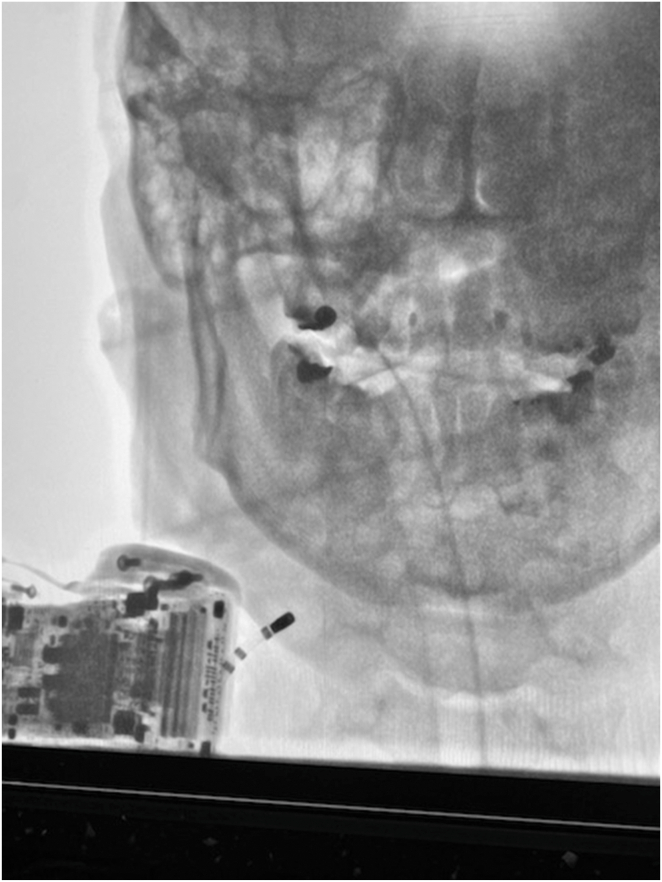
Ultrasound assessment of vagus nerve position in relation to the course of the internal jugular vein.
